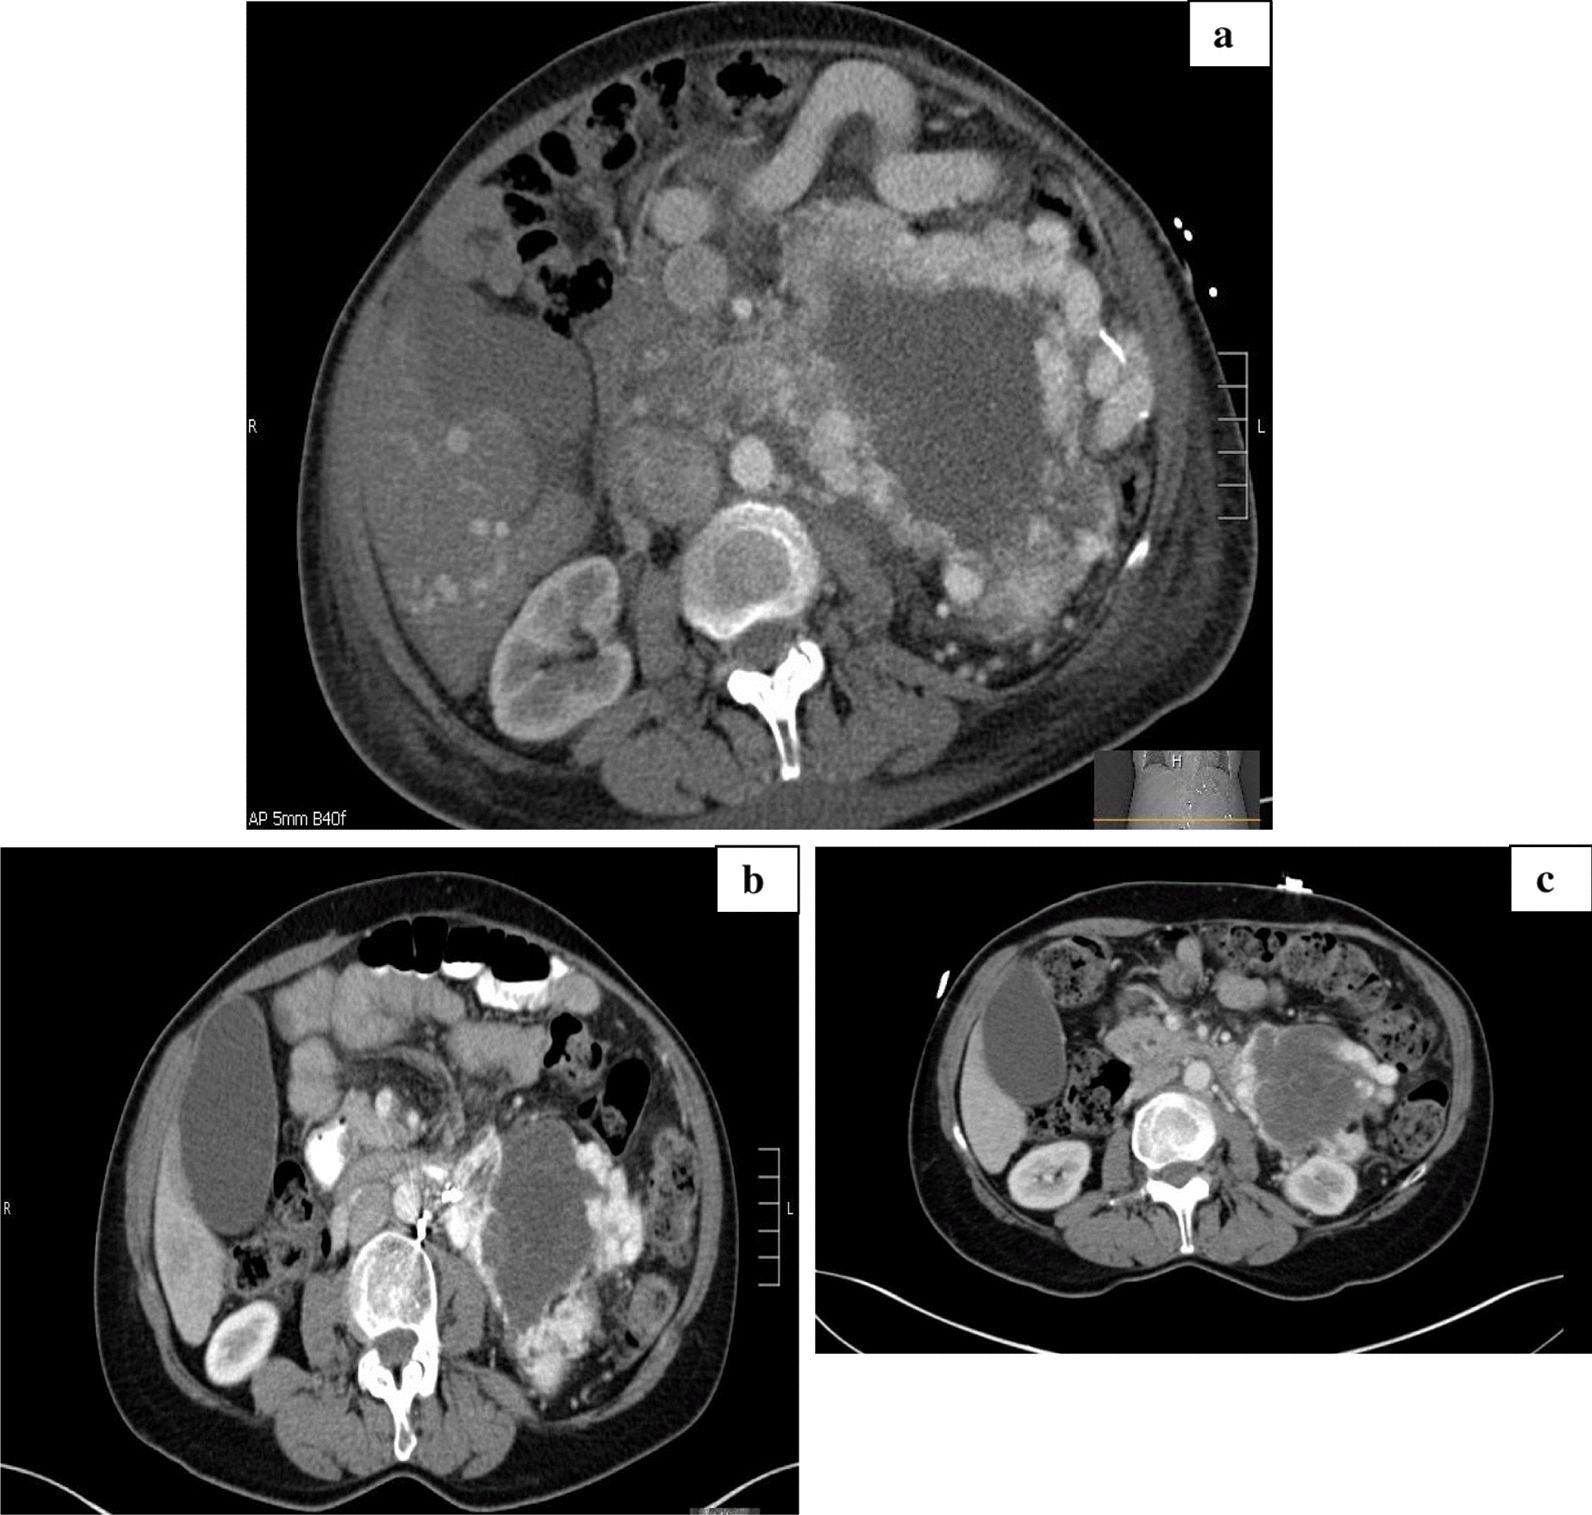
a Computed tomography scan pre-immunotherapy treatment, b CT restage IO × 5 months, and c CT restage IO × 8 months
